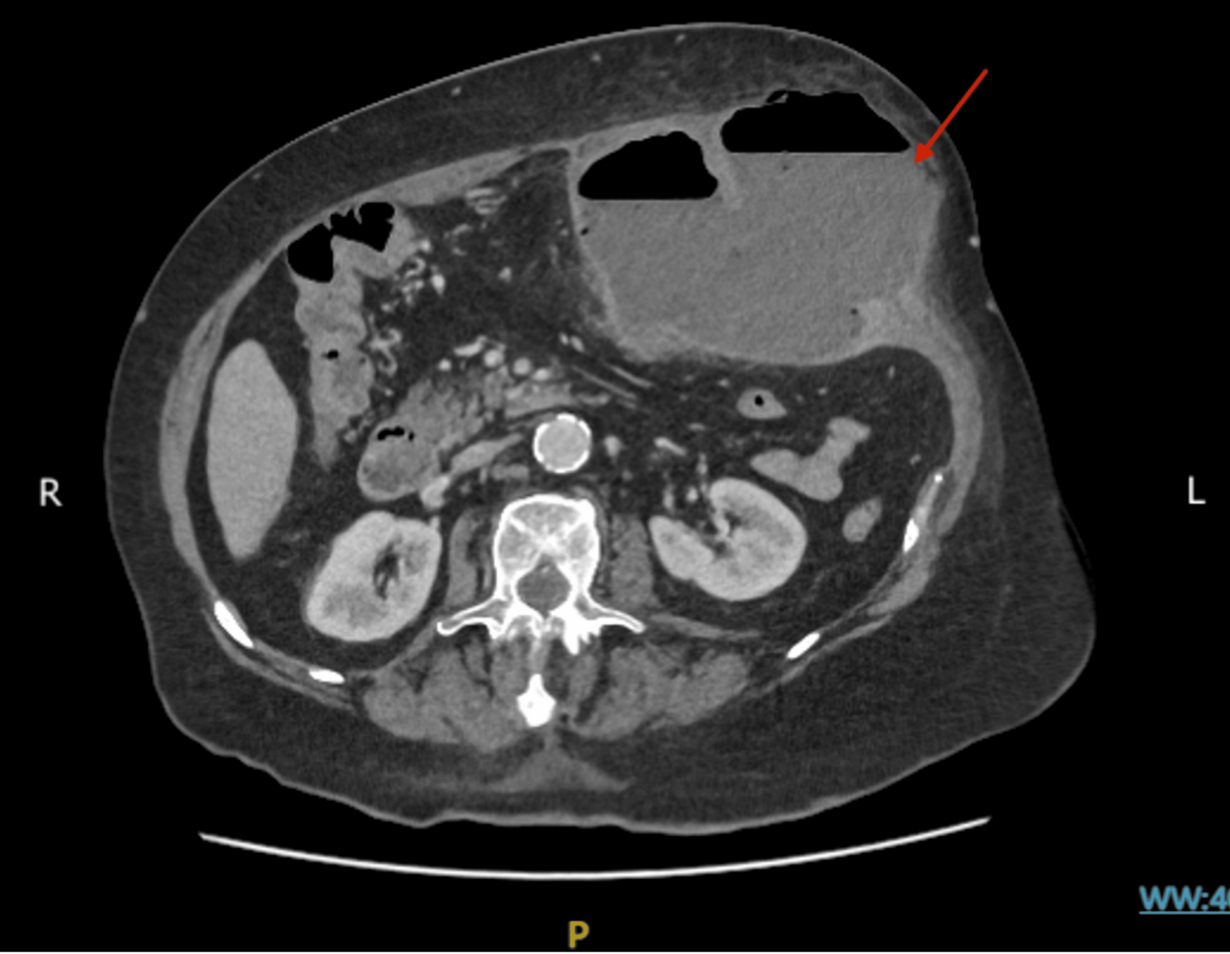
Axial contrast-enhanced CT scan showing an infected collection in the left anterior abdominal wall (red arrow).
